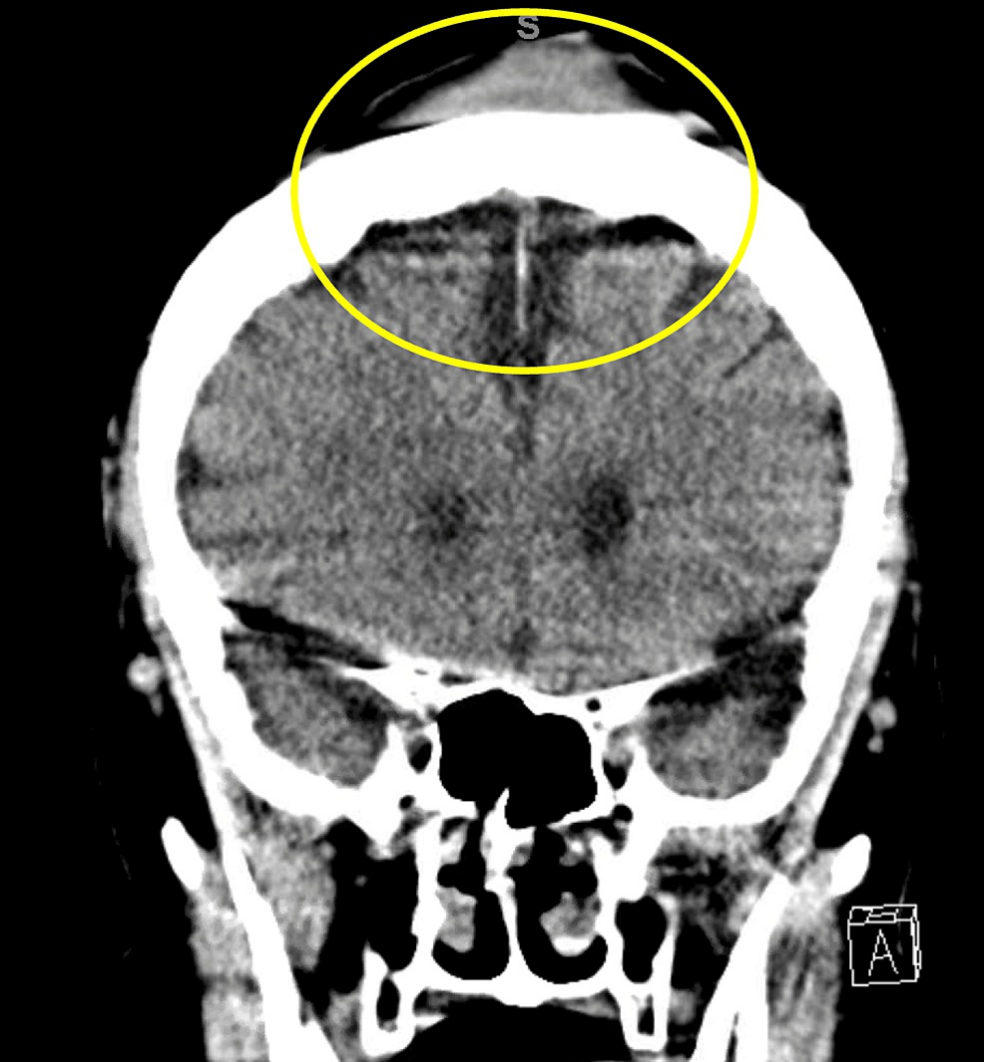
CT of the head The yellow circle highlights the area in which a vertex hematoma can be visualized, likely due to the level-ground fall that the patient sustained. There are no other abnormalities in this study.
